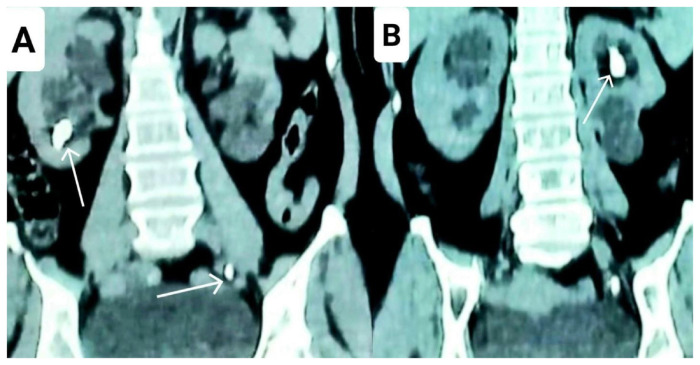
Plain CT kidney-ureter-bladder (KUB) coronal view. A: Calculus measuring 2.2 cm indicated by a white arrow is visible in the lower pole of the lower moiety of the right duplex kidney and another calculus measuring 1 cm indicated by a white arrow is visible in the line of the left ureter at the level of pelvic brim. B: Calculus measuring 1.5 cm indicated by a white arrow is visible in the pelvis of the upper moiety of the left duplex kidney.
